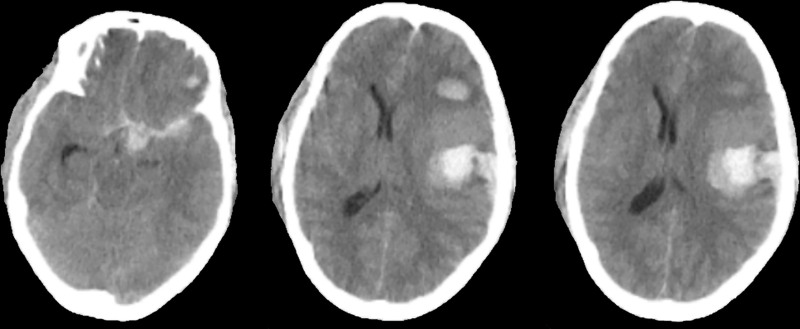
Brain axial computed tomography (CT) scan of the patient prior to surgery.
